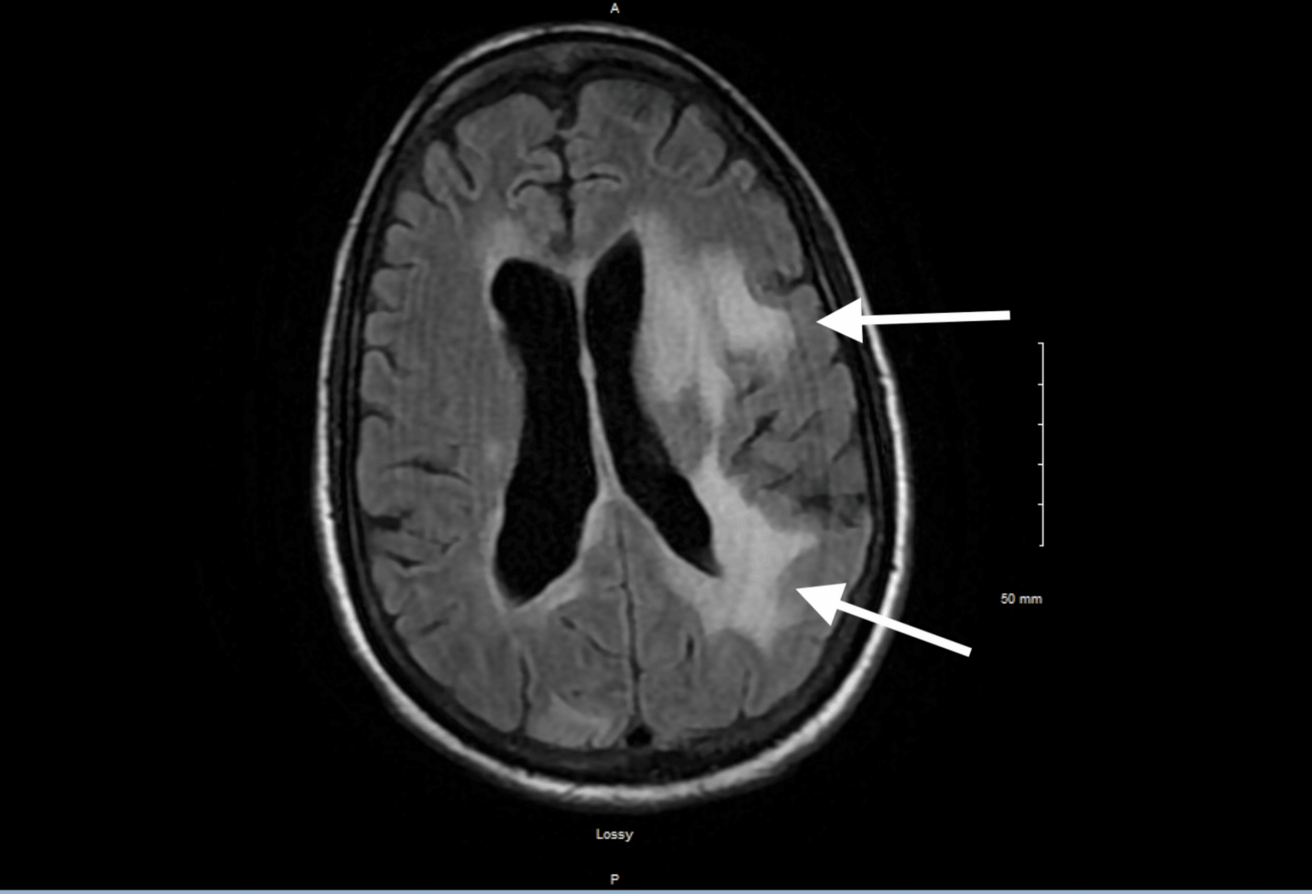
Contrast-Enhanced MRI of the Brain MRI of the brain with contrast shows multiple enhancing lesions in the supratentorial brain and left cerebellum (white arrows), predominantly in periventricular distribution.
